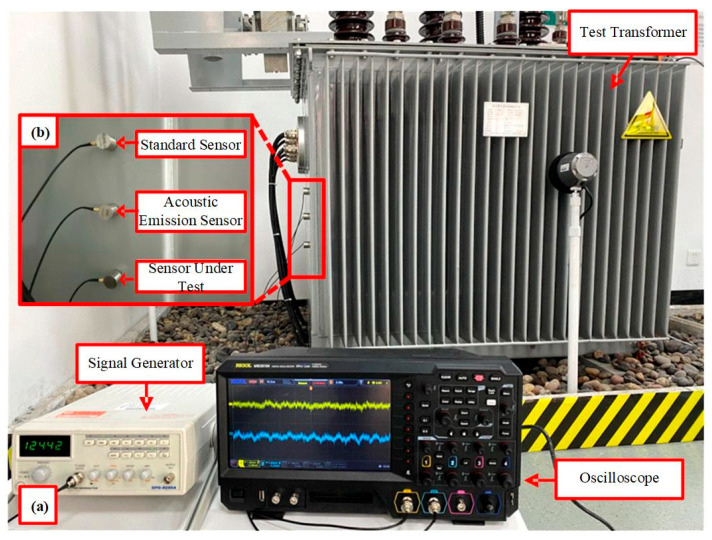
(a) Transformer partial discharge ultrasonic sensor verification platform. (b) Enlarged view of sensor arrangement.
